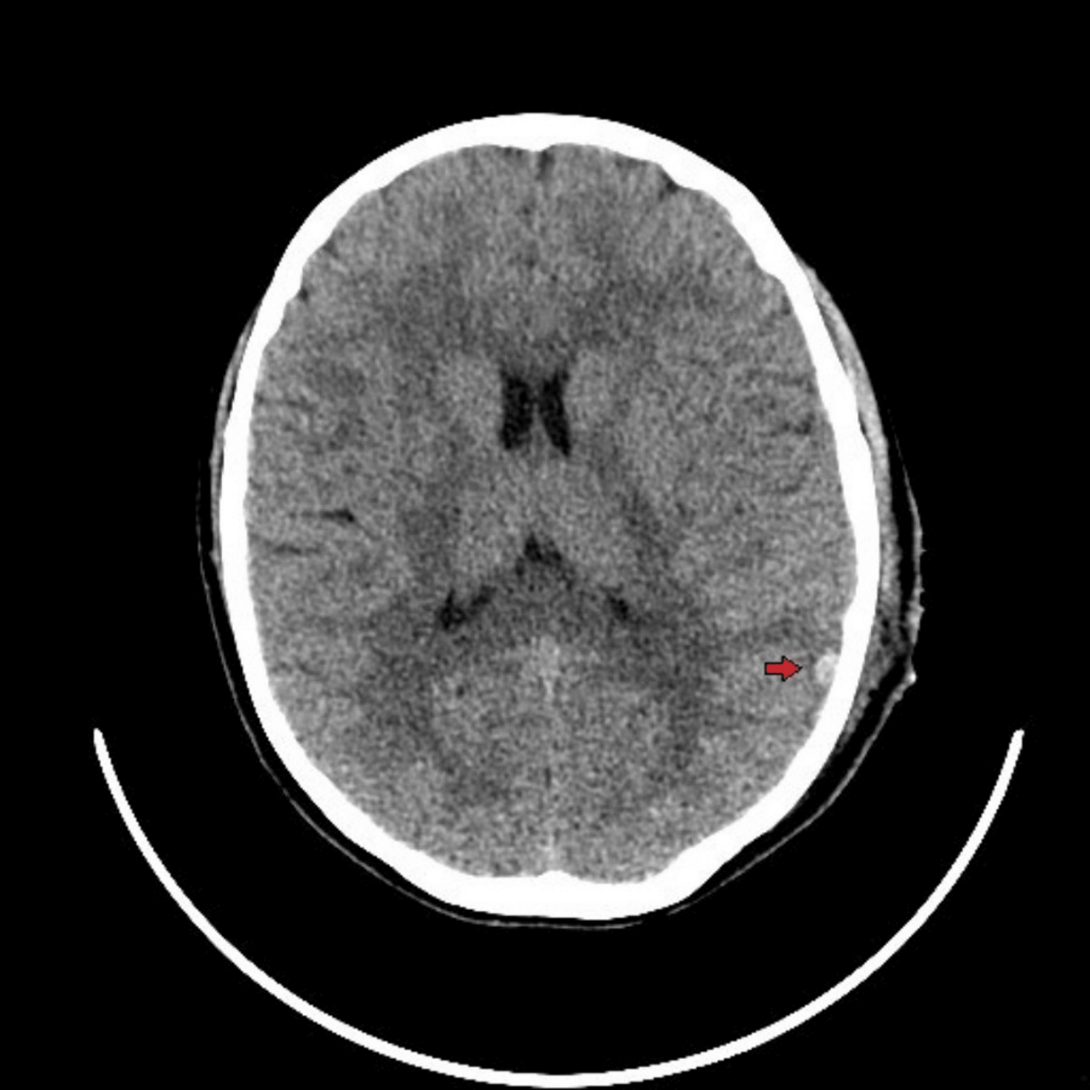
CT head of patient 4 Axial non-contrast CT of the head showing a small left parietal contusion (red arrow). On neurological examination, the patient presented as awake, alert, and oriented to person, place, and time, with fluent speech, pupils 3 mm in diameter and bilaterally reactive, and motor function with full strength in all extremities. CT: computed tomography
